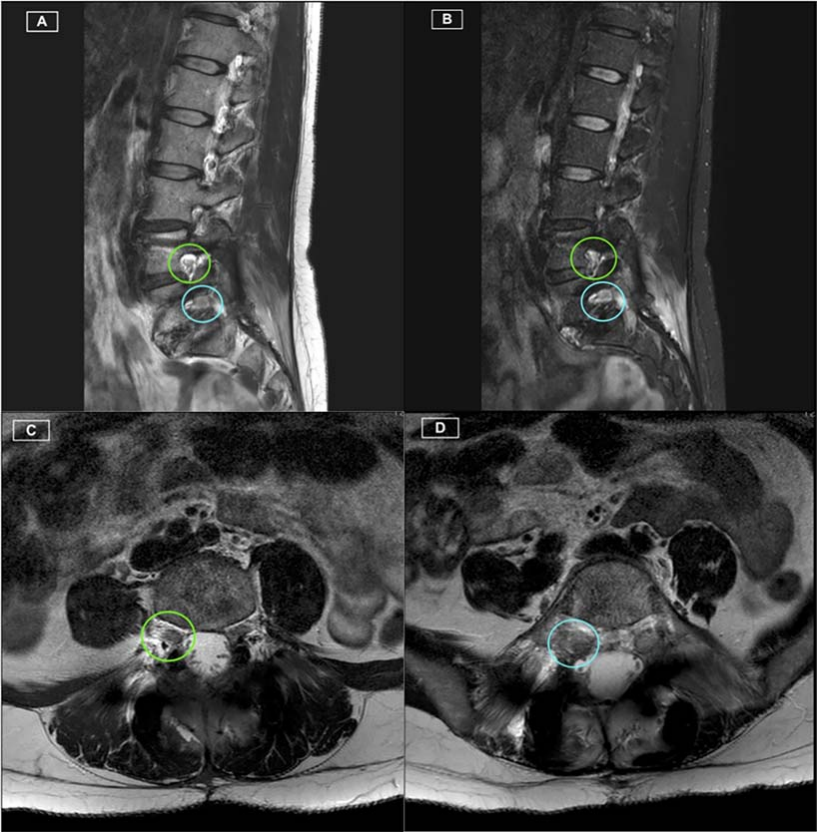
Postoperative MRI Lumbar spine. (A) Right-sided parasagittal slice of T2 Sequence MRI. Right L4/5 foramen (superior) and Right L5/S1 foramen (inferior) have been highlighted, with excellent definition of the Right L4 and L5 nerve roots. (B) Right-sided parasagittal slice of Short Tau Inversion Recovery (STIR) Sequence MRI. Exiting L5 nerve root is well visualised, with evident perineural thickening. (C) Axial slice of T2 Sequence MRI at the level of the L4/5 Foramen. The Right L4 nerve root is identified with excellent visualisation. (D) Axial slice of T2 Sequence MRI at the level of the L5/S1 Foramen. The Right L5 nerve root is identified with excellent visualisation.
